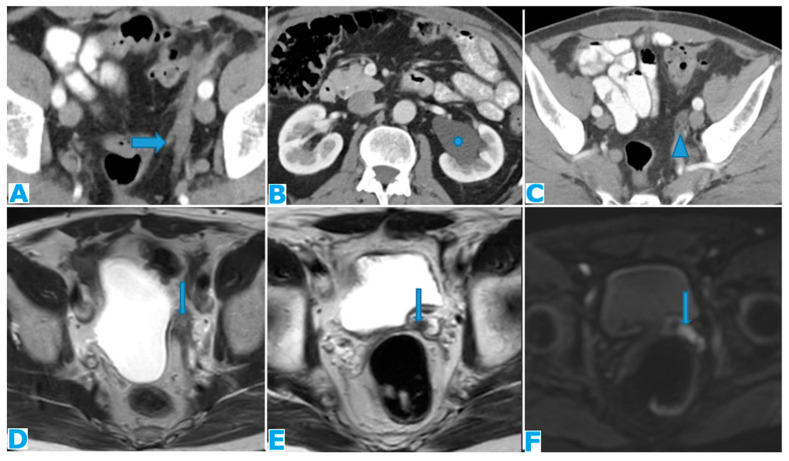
Axial CE-CT (A–C). Axial T2WI (D,E); DWI (F). PC from mucinous adenocarcinoma of the appendix: Notice the peritoneal deposit within the left lateral pelvis (arrow in (A)) as an elongated soft tissue mass. The patient presented with a left ureterohydronephrosis (* in (B)) due to the pelvic deposit, which obstructed the ureter (arrowhead in (C)). Paravesical spaces are peritoneal recesses that cover, on each side, the distal ureter, the seminal vesicle, and the deferent duct. Note the deposit within the left paravesical space and how it obstructs the left ureter (arrow in (C)). The deposit also follows the course of the left deferent duct (arrow in (E,F)).
